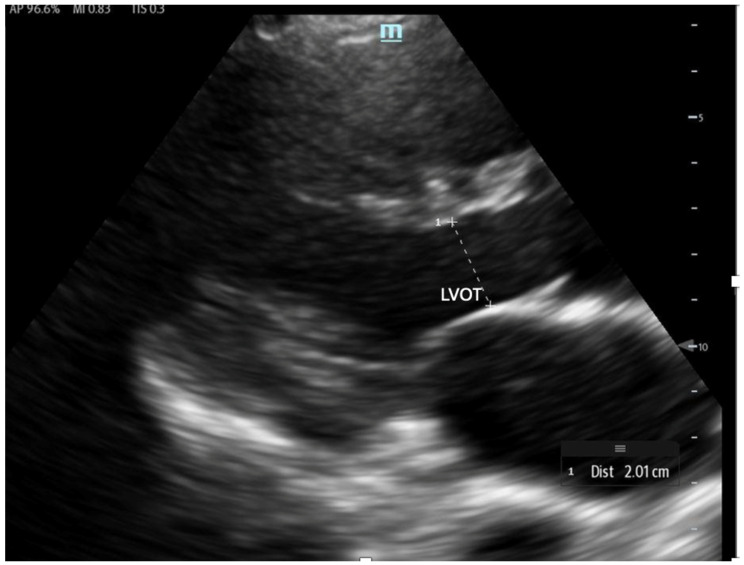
The LVOT diameter measured below the opening of the aortic valve in a zoomed-in parasternal long axis to be incorporated into the LVOT area formula.
